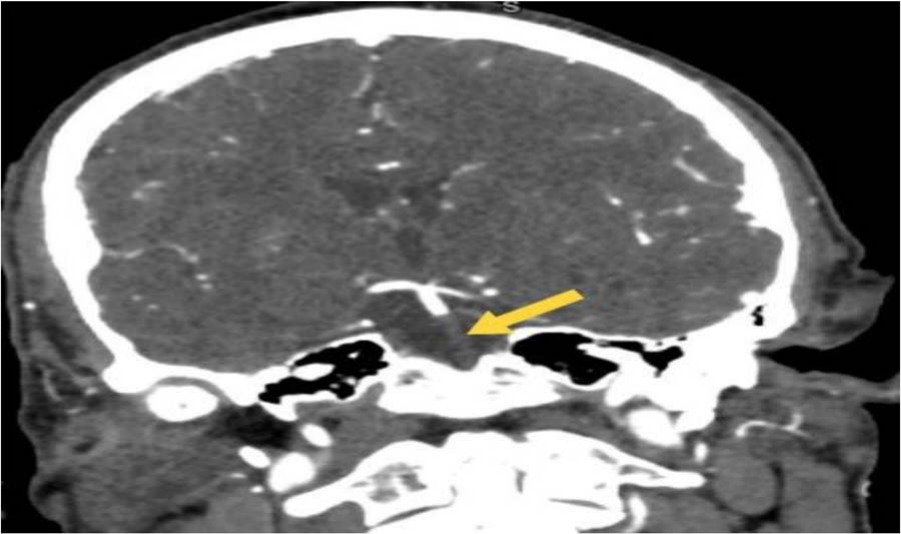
Coronal CTA image of the brain demonstrating abrupt loss of contrast opacification at the origin of the basilar artery (arrow), confirming the presence of a proximal basilar artery occlusion.
